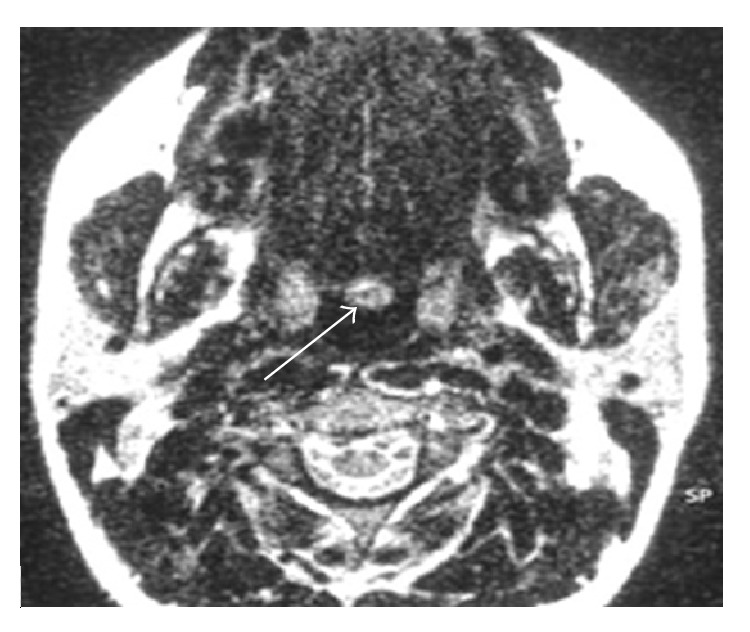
Axial MRI: lingual thyroid.
